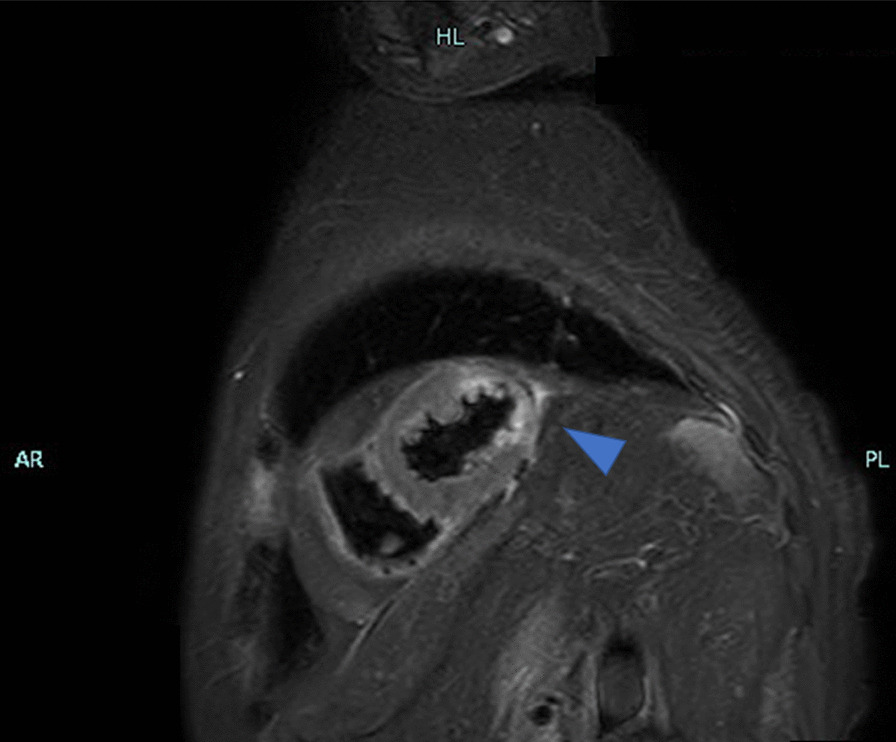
Contrast-enhanced MRI image on the 33. T2WI showed high signal mainly subintima (partially penetrating) from the lateral wall to the posterior wall, which coincides with the posterior aspect of the heart (triangle)
